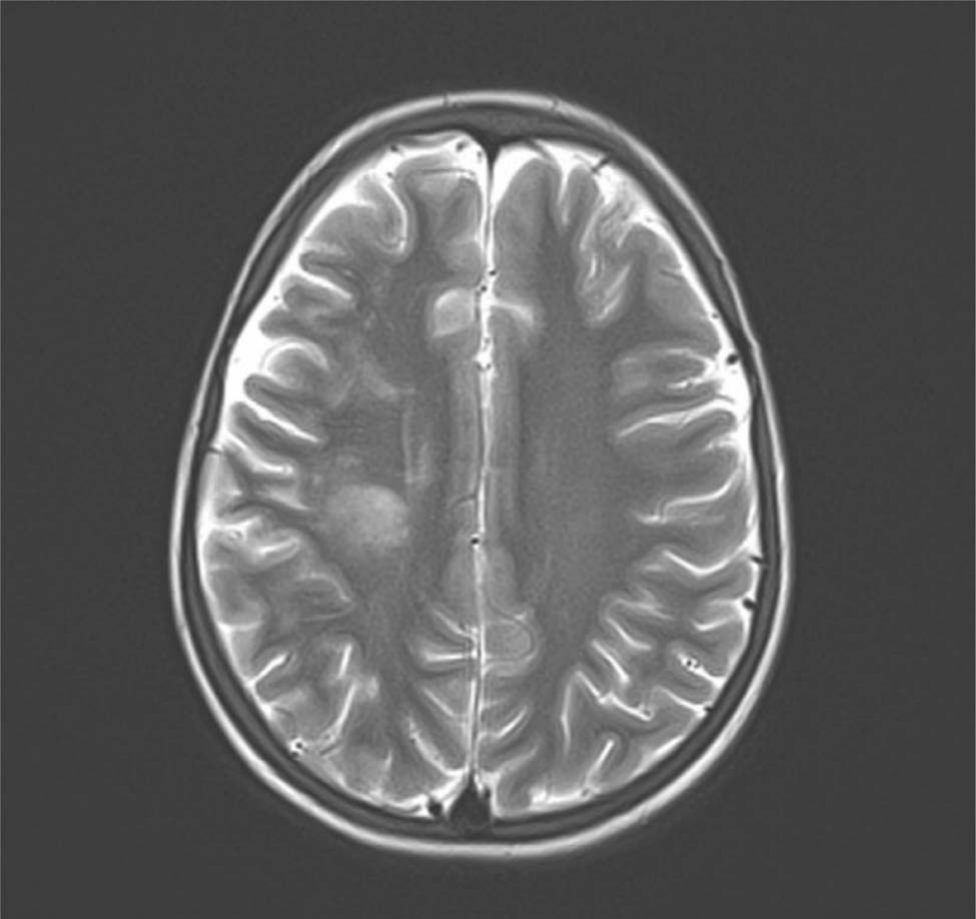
T2-weighted brain MRI showing right sub-acute temporoparietal infarction.
